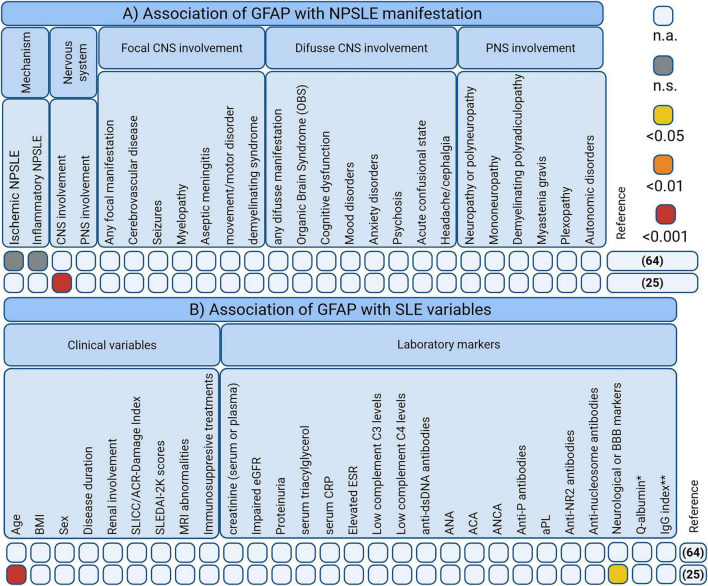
Comparative map of clinical variables related to GFAP serum levels. (A) Association of GFAP with NPSLE manifestation and (B) Association of GFAP with SLE demographic and clinical variables. This figure provides a comparative overview of how different studies, using diverse statistical approaches, have examined the relationship between serum GFAP and clinical variables. Readers can interpret the grid by following the color coding, which indicates statistical significance: Red, *p* < 0.001; Orange, *p* < 0.01; Yellow, *p* < 0.05; Gray, not significant; Blue, not assessed. In this way, the figure highlights both consistently reported associations and underexplored aspects. Further details of each association are described in the main text. GFAP, glial fibrillary acidic protein; NPSLE, neuropsychiatric systemic lupus erythematosus; CNS, central nervous system; PNS, peripheral nervous system; BMI, body mass index; SLICC/; ACR, systemic lupus international collaborating clinics/american college of rheumatology; SLEDAI-2K, systemic lupus erythematosus disease activity index 2000; MRI, magnetic resonance imaging; eGFR, estimated glomerular filtration rate; CRP, C-reactive Protein; ESR, erythrocyte sedimentation Rate; anti-dsDNA antibodies, anti-double-stranded deoxyribonucleic acid antibodies; ANA, antinuclear antibodies; ACA anticentromere antibodies; ANCA, anti-neutrophil cytoplasmic antibodies; Anti-P, anti-ribosomal P; aPL, antiphospholipid antibodies. Q-albumin, quotient albumin*, is the ratio between CSF/Serum albumin, higher Q-albumin indicates BBB disruption. IgG index [(CSF/S IgG ratio)/(CSF/S albumin ratio)], higher IgG index indicates IgG CNS production. Created with BioRender.com.
